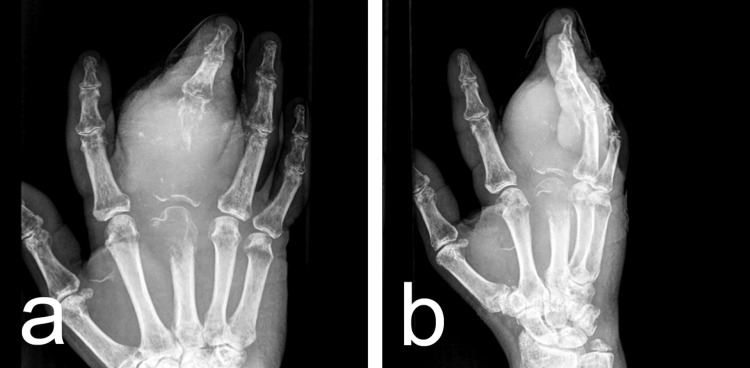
An initial X-ray of the swollen hand revealed a large soft tissue mass surrounding the head of the third metacarpal bone, as well as the proximal and middle phalanges of the third finger, accompanied by bone lysis.
